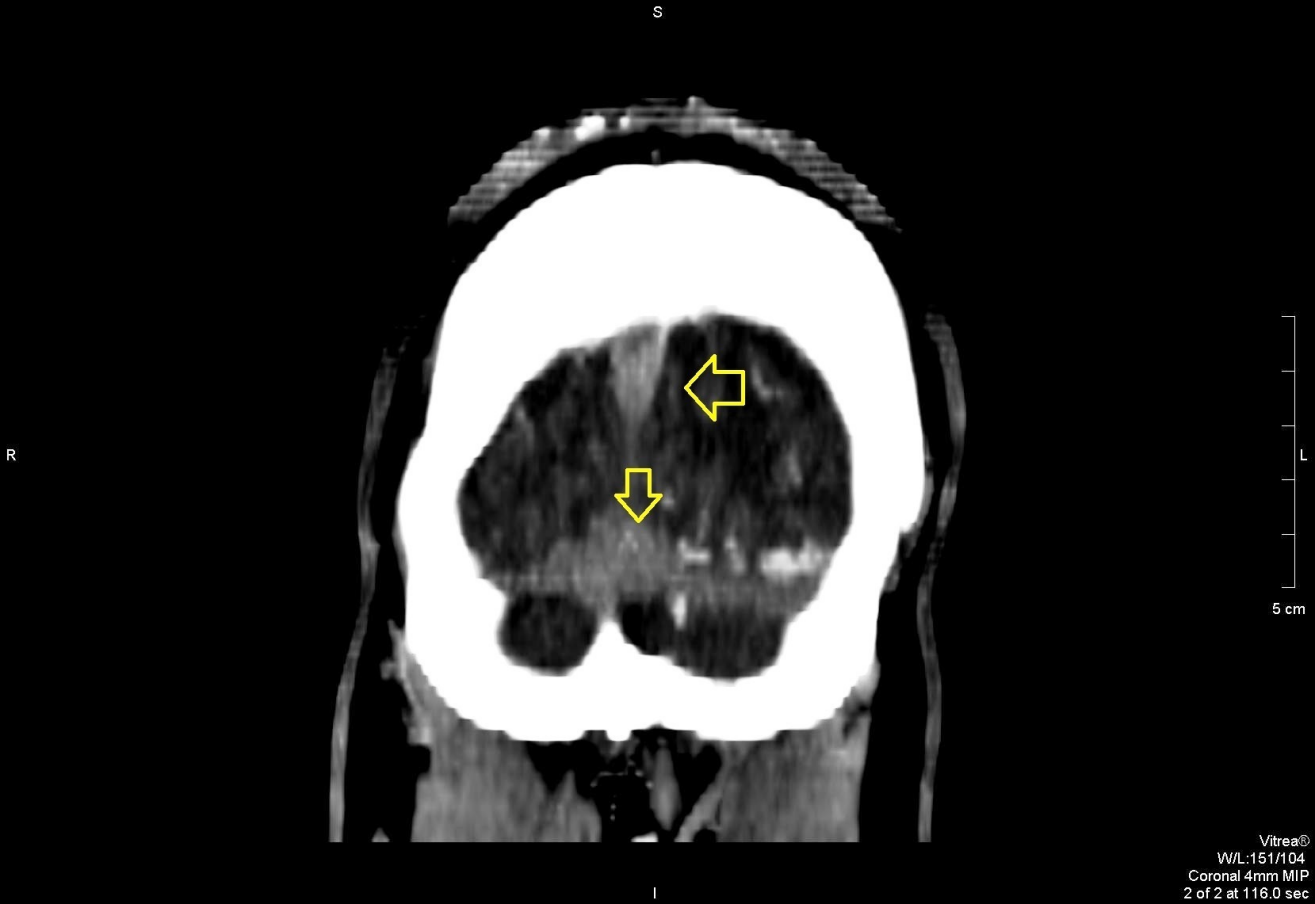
Coronal view on contrast CT scan showing superior sagittal sinus blockage and extensive thrombosis at the confluences of the sinuses CT: computed tomography
